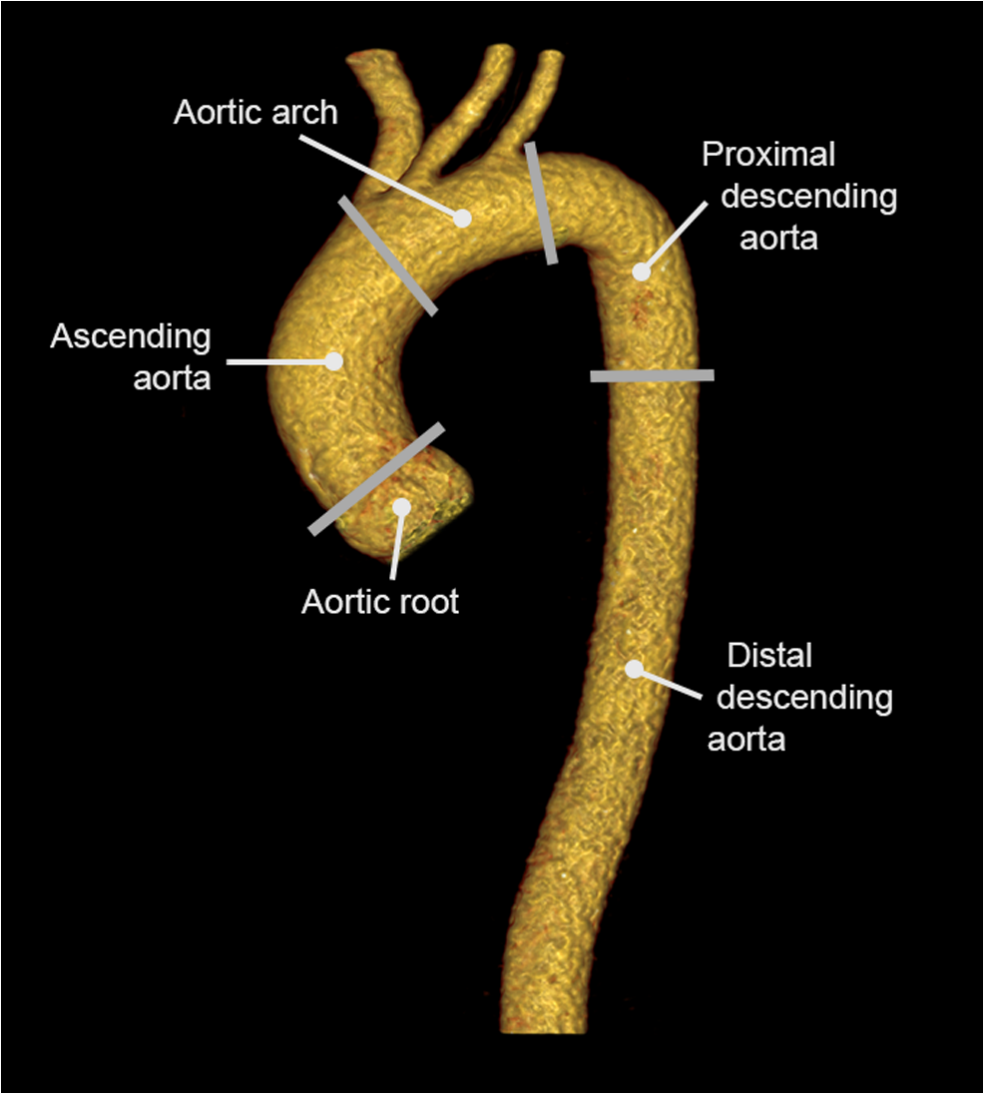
Three-dimensional CT reconstruction of a healthy thoracic aorta. The ascending aorta runs from the sinotubular junction until the first branch vessel (brachiocephalic trunk), while the aortic arch is defined as the segment that contains the three branch vessels. The descending thoracic aorta is divided into two parts: a proximal part (from the left subclavian artery to the level of the pulmonary artery) and a distal part (from the pulmonary artery to the diaphragm)
